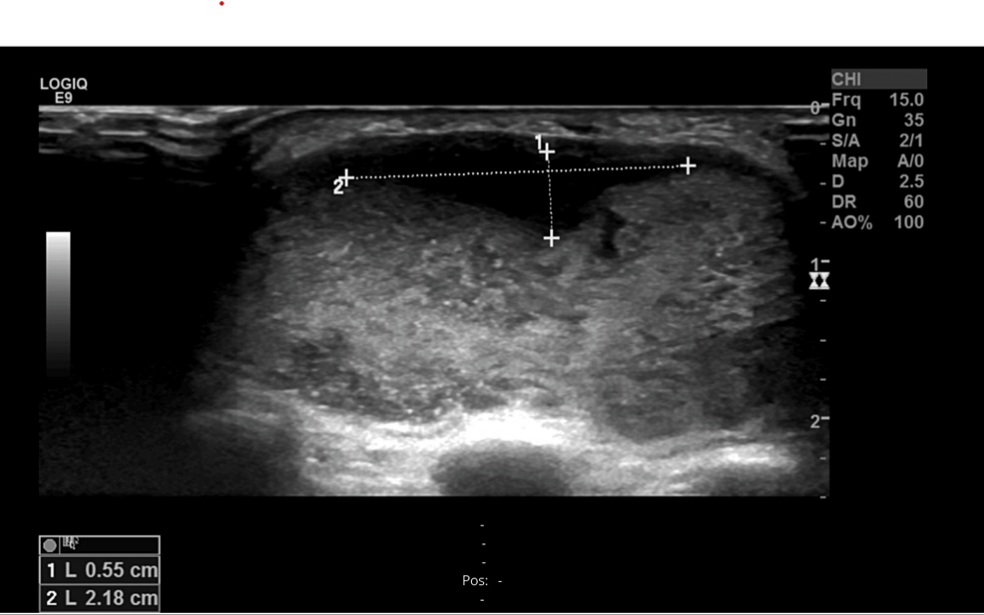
An ultrasound of the dorsal side of the left wrist. The lines show that the accumulation, which contained an inhomogeneous liquid, measured 2 cm X 0.5 cm X 6.5 cm
